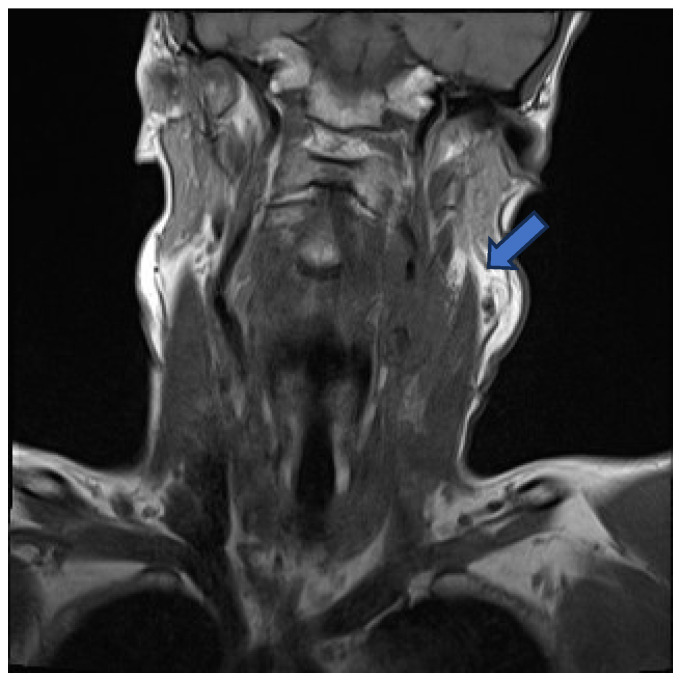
MRI—mass encasing the left common carotid, carotid bulb, and internal carotid arteries (blue arrow).
